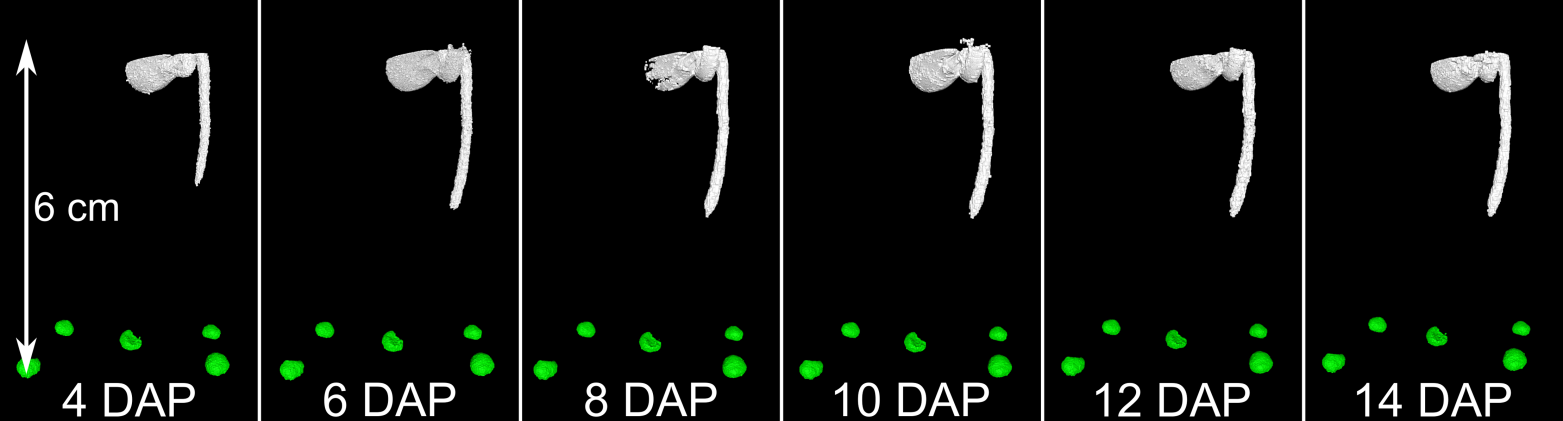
Time series of a taproot from *Vicia faba* visualised every second day by X-ray CT; scanning settings were chosen for best image quality; lethal influence on root tissue can be seen, as the root did not grow further within 14 days.
